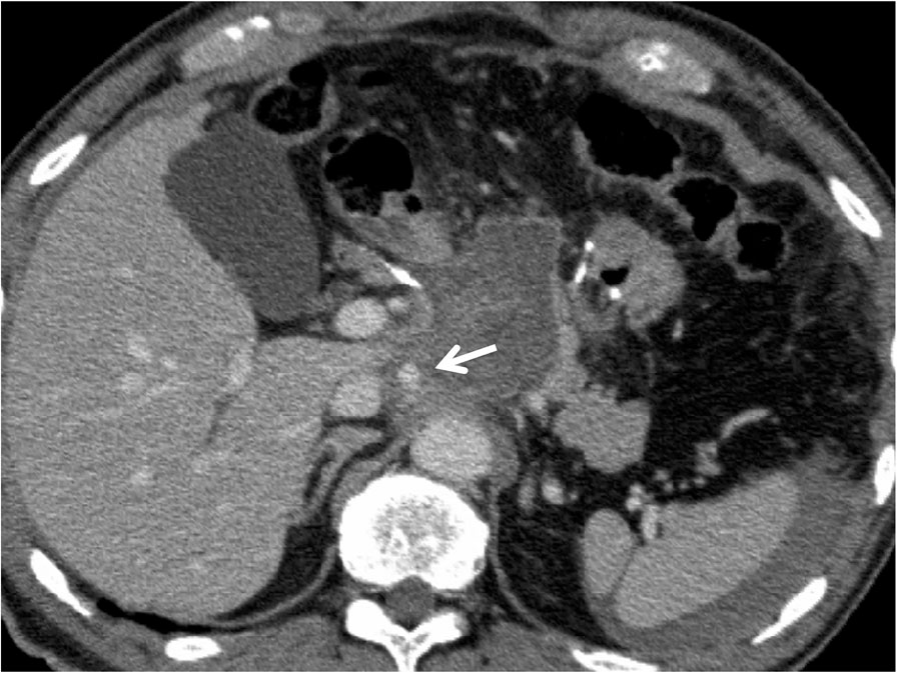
CT revealed a 60 × 70-mm hematoma and a 10-mm pseudoaneurysm (arrow) in the RIPA
